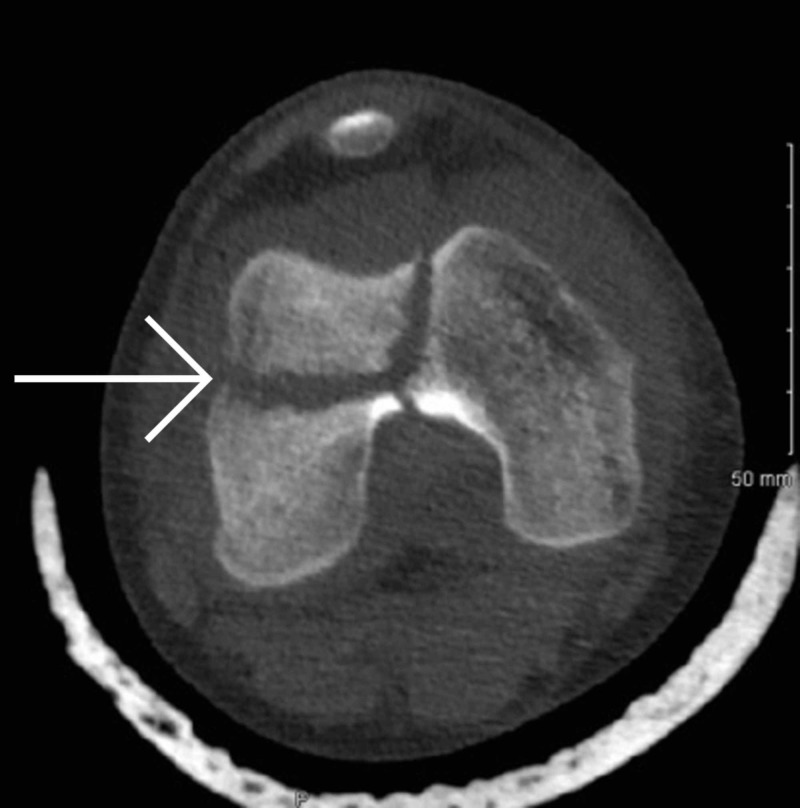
Axial view of computed tomography scan of right distal femur post-injury
